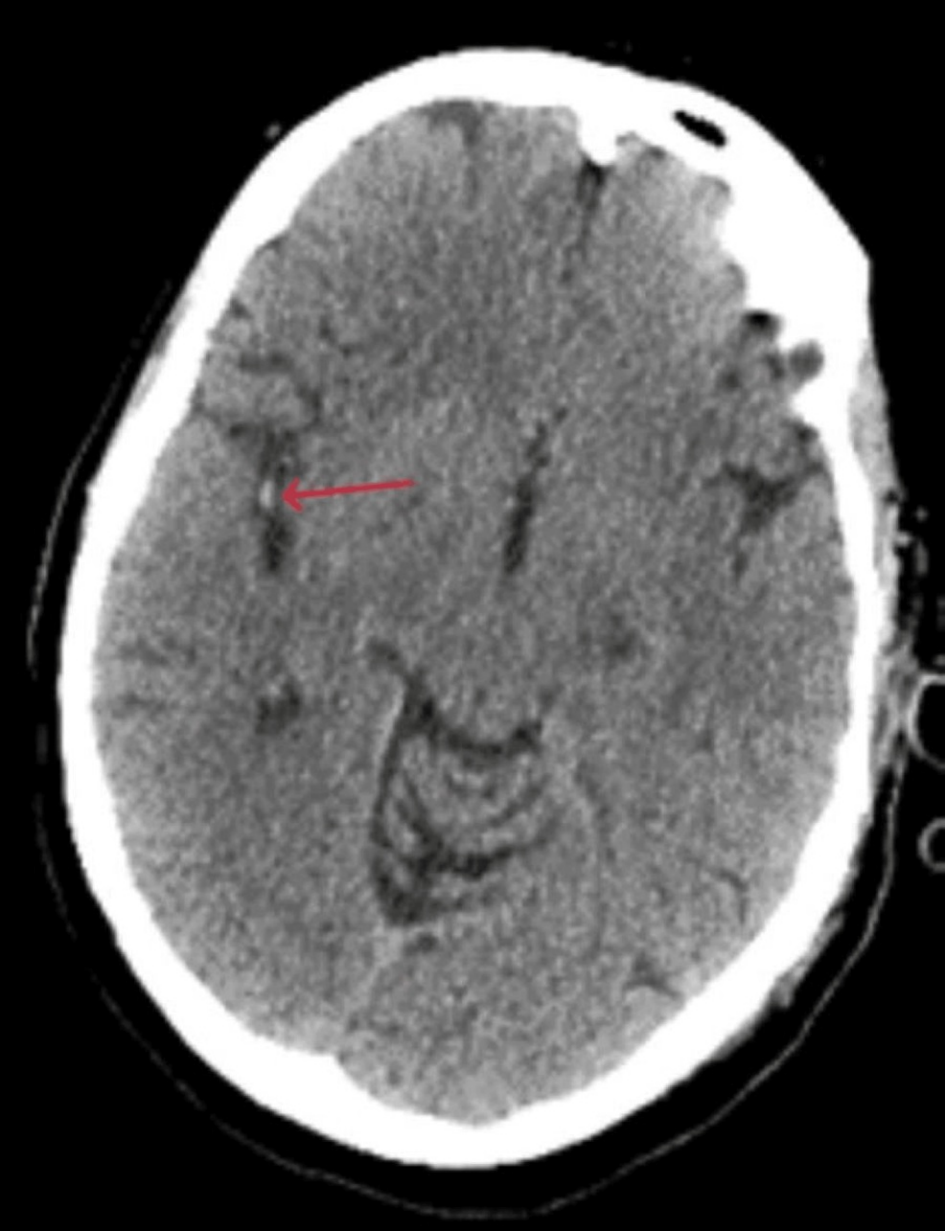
Axial brain CT showing right M2 division hyperdensity (red arrow) suggestive of arterial occlusion
